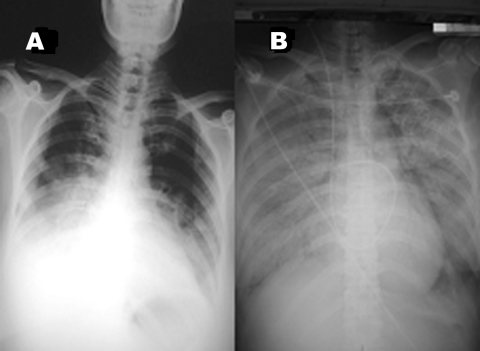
A) Chest radiograph on hospital day 5 at referring hospital shows patchy infiltration at bilateral lower lung fields. B) Chest radiograph upon admission to our hospital (24 hours later) shows rapidly progressive pneumonia in both lung fields, compatible with adult respiratory distress syndrome.
